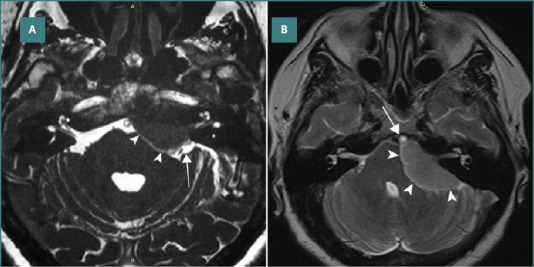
MRI images depict the relation between skull base meningiomas and the subarachnoid cisterns in the posterior fossa. A, shows a meningioma arising at the level of the prepontine cistern. The arachnoid membrane is pushed posteriorly (arrowheads). The cerebellopontine cistern and the neurovascular elements within are pushed laterally and inferiorly (arrow). B, shows a meningioma arising at the level of the cerebellopontine cistern. The arachnoid membrane is pushed medially (arrowheads), and no cleavage plane exists between a tumor and the neurovascular elements within this cistern. The prepontine cistern and the neurovascular elements within are pushed medially (arrow).
